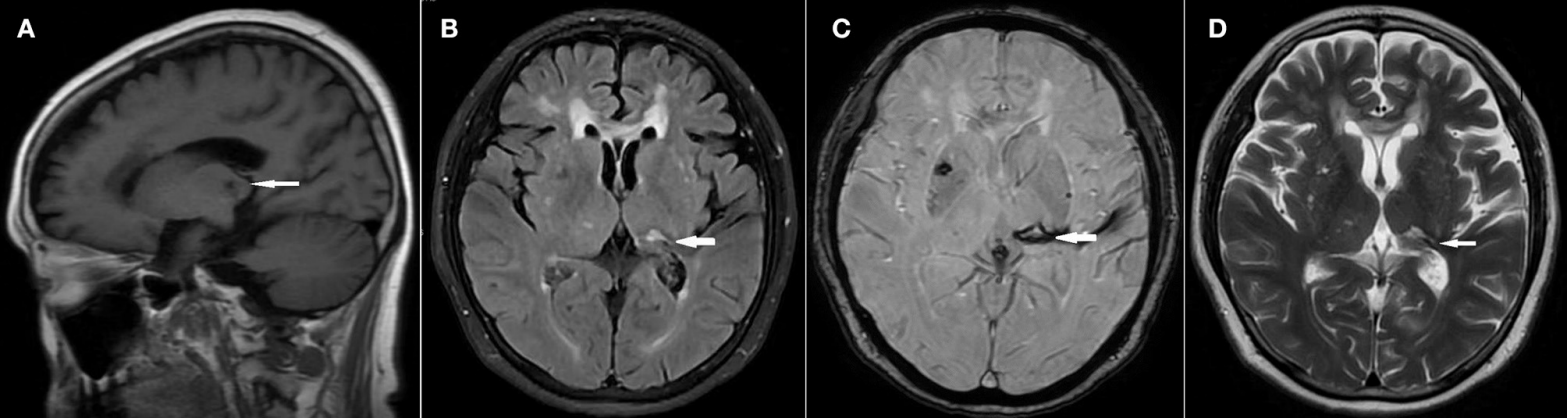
MRI without contrast showed ischemic stroke. The arrows indicated lesion of malacic at the left thalamus. (A) Saggital T1WI. (B) Axial T2 FLAIR. (C) 3D SWAN. (D) Axial T2WI.
